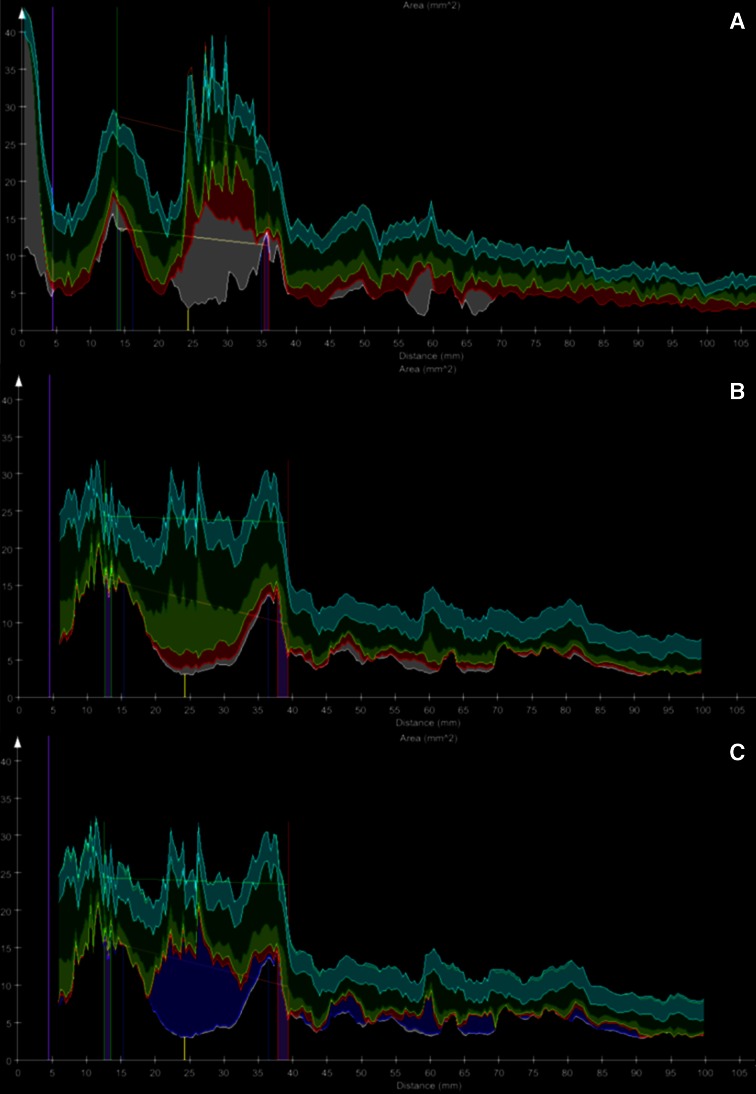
Quantification of plaque volumes along the vessel. a An example of a coronary lesion in CTA. The X-axis represents the distance from the coronary ostium in mm. The Y-axis represents the area of either the lumen (*lower part of graph*) or the vessel wall (*upper part of graph*) in mm^2^. The part between the two graphs shows the plaque constitution using a *color code*. In the corresponding VH data is shown in b and in c the results with the quantified *shadows in blue*. The enhanced quantification has better correspondence with the CTA analysis after adding the quantified *shadow areas* to the DC
